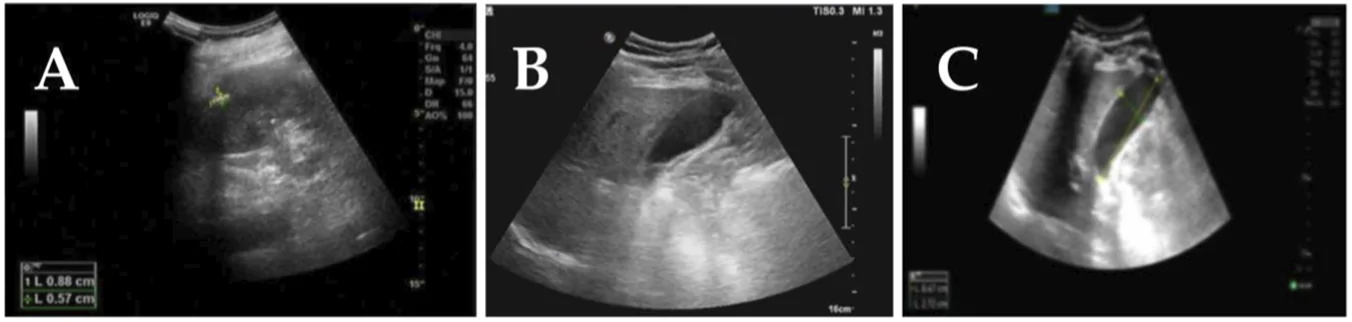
Abdominal Ultrasound results for the patient (A) September 2024: The gallbladder measured 90 mm × 39 mm with a wall thickness of 4 mm and irregular mural surface; fine punctate intraluminal deposits can be seen. (B) November 2025: The gallbladder is normal in size with mural thickening measuring 10 mm, demonstrating the double-wall sign. (C) July 2026: The gallbladder measured 85 mm × 27 mm with a mural thickness of 2 mm and a smooth wall.
